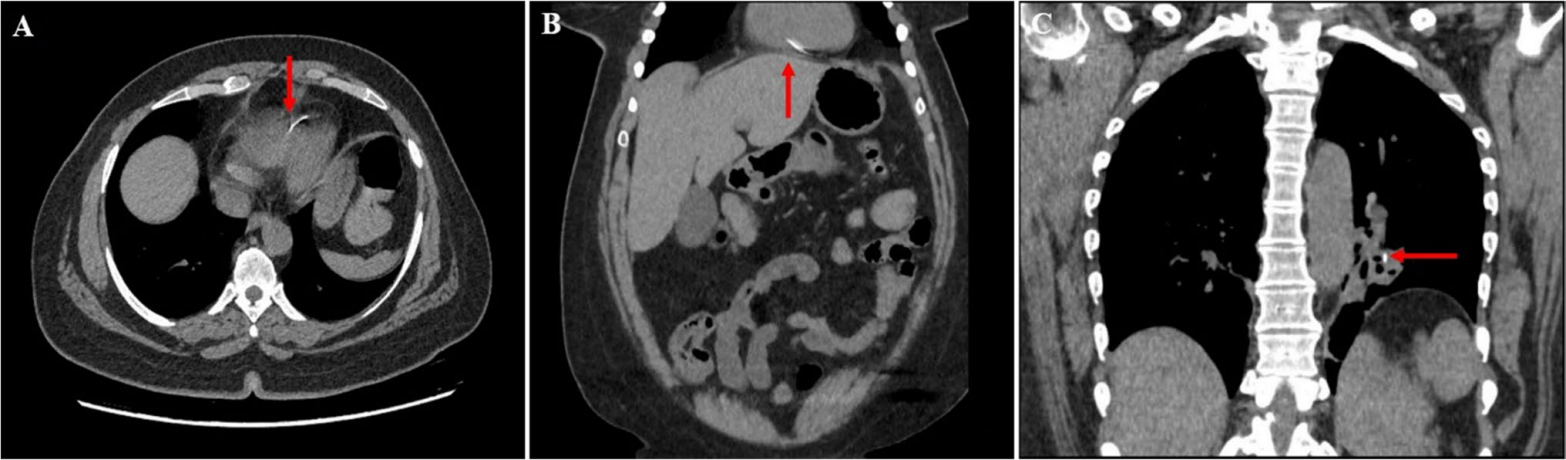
A, B Axial and coronal reconstruction of computed tomography (CT) showing linear density noted in the right ventricle. C Coronal CT-chest demonstrating inferior vena cava filter strut embolized to left inferior pulmonary artery segmental branch
